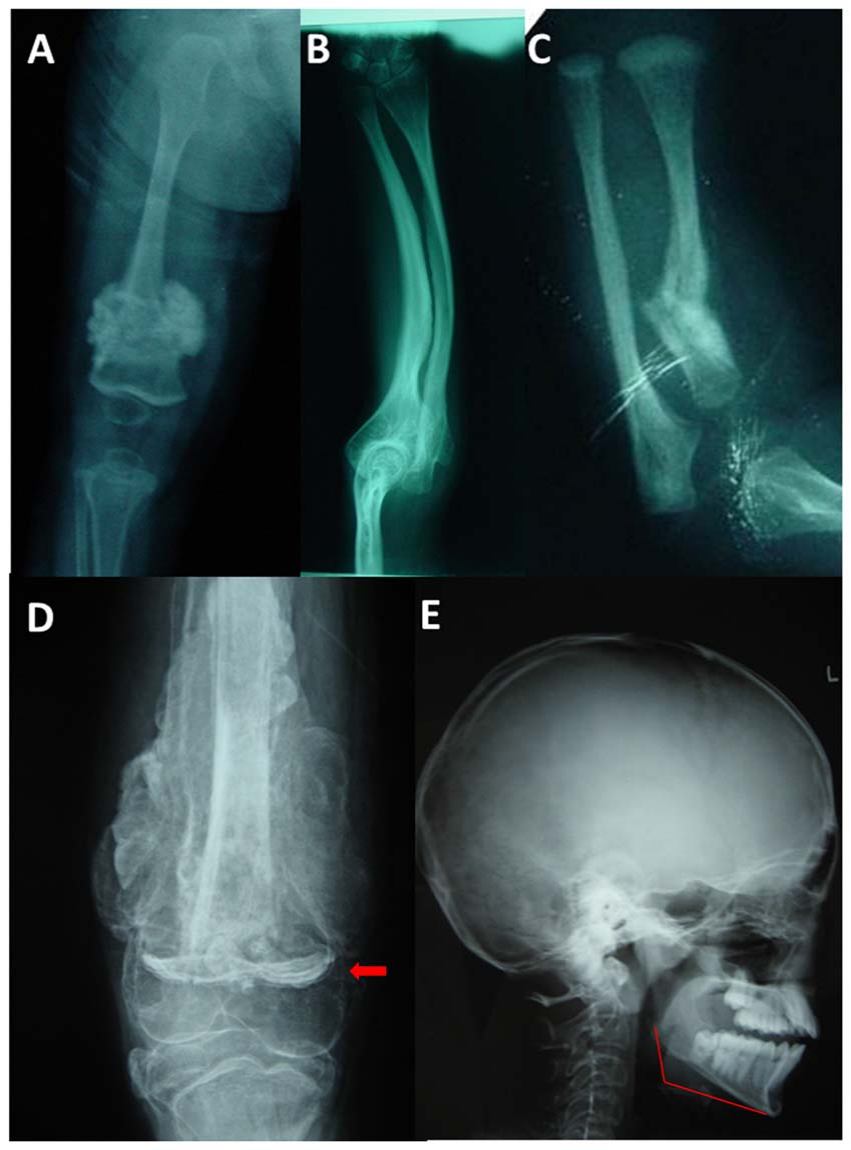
Typical radiographic manifestations. (A) Radiography of the proband of family 1 revealed an irregular radiodense mass arising from the right femur and widened metaphyses with metaphyseal dense bands. (B) Radiography of the affect mother of family 1 revealed calcification of the forearm interosseous membrane and radial-head dislocation. (C) Radiography of the proband of family 3 revealed metaphyseal dense bands of distal radial but no calcification of the forearm interosseous membrane or radial-head dislocation. (D) Radiography of the proband of family 4 revealed an irregular radiodense mass arising from the left femur and “zebra” lines (arrow) induced by intravenous ibandronate. (E) Skull radiography of the proband of family 4 revealed Wormian bones and mandibular hypoplasia (the drawn line represents the normal contour).
